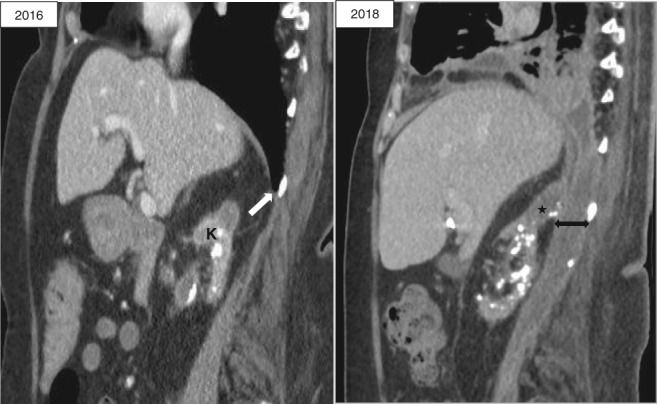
Sagittal computed tomography images of 2016 showing right posterolateral diaphragmatic defect (white arrow) and atrophic kidney (K), allowing transit of perinephric and psoas abscess (star) via the pre‐existing defect (left–right arrow) into pleural cavity in 2018.
